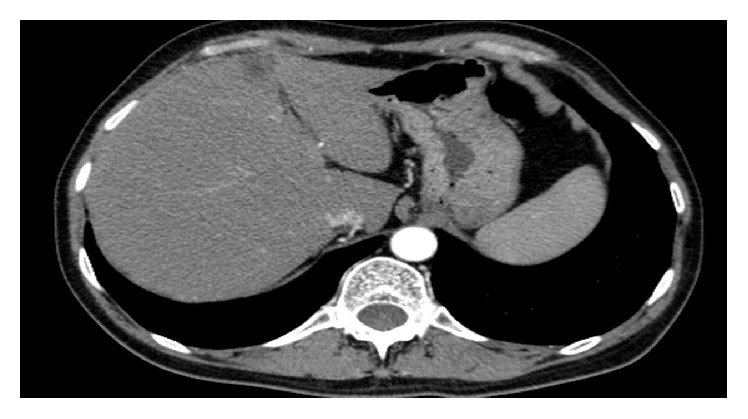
Computed tomography (CT) revealed a thickened gastric wall mainly involving the pyloric region.
